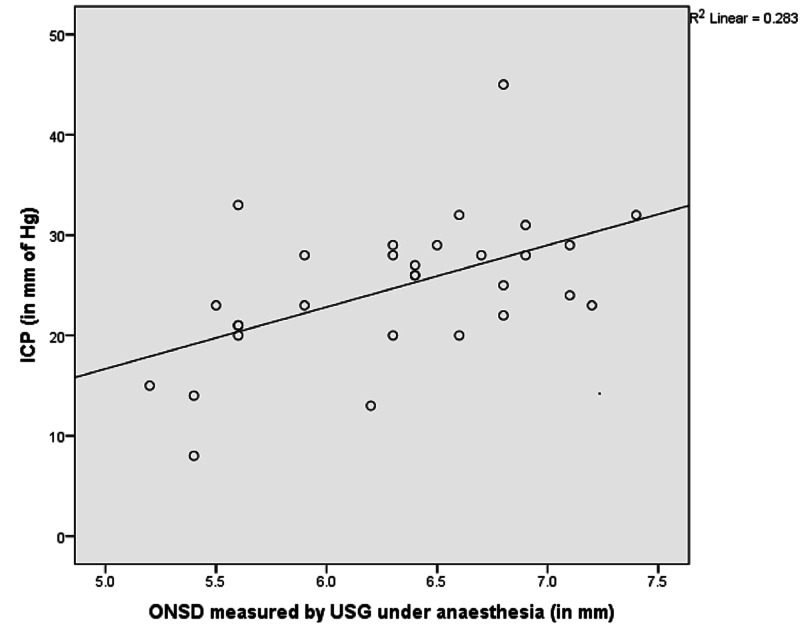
Scatter plot showing correlation between ONSD measured by ultrasonography and the ICP ONSD = optical nerve sheath diameter; ICP = intracranial pressure; USG = ultrasonography
